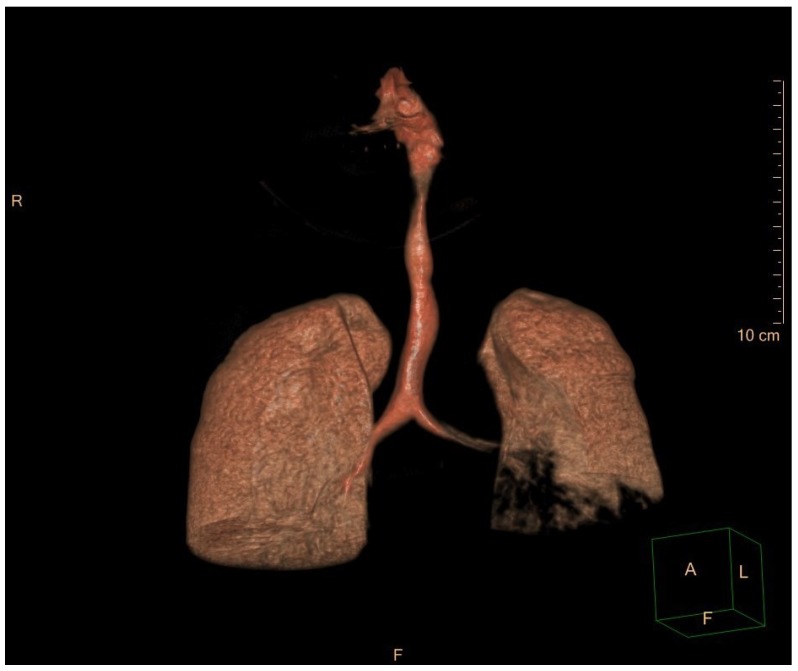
3D CT scan dataset for multiplanar reconstruction shows marked tracheal narrowing and tortuosity.
